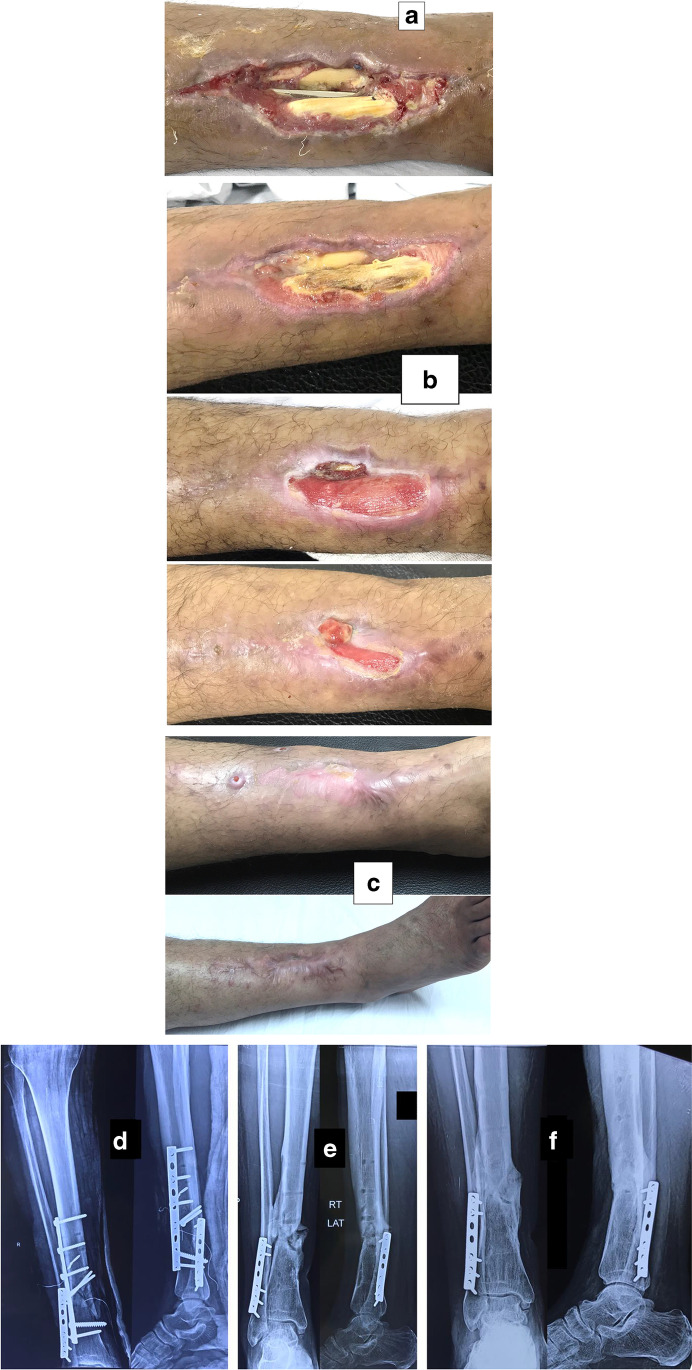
A 53-year-old diabetic smoker patient presented with a postoperative complicated infected wound in the distal part of his right leg following open reduction and internal fixation with plate and screws of closed distal tibial fracture. a Clinical photo at presentation with bone, plate and tendon exposure. b Clinical photos of different stages of wound repair. c Residual sinuses before implant removal (top) and complete bone coverage with dry scar after 39 months of follow-up (bottom). d, e, f Radiographs at presentation, after implant removal, and at the last follow-up
